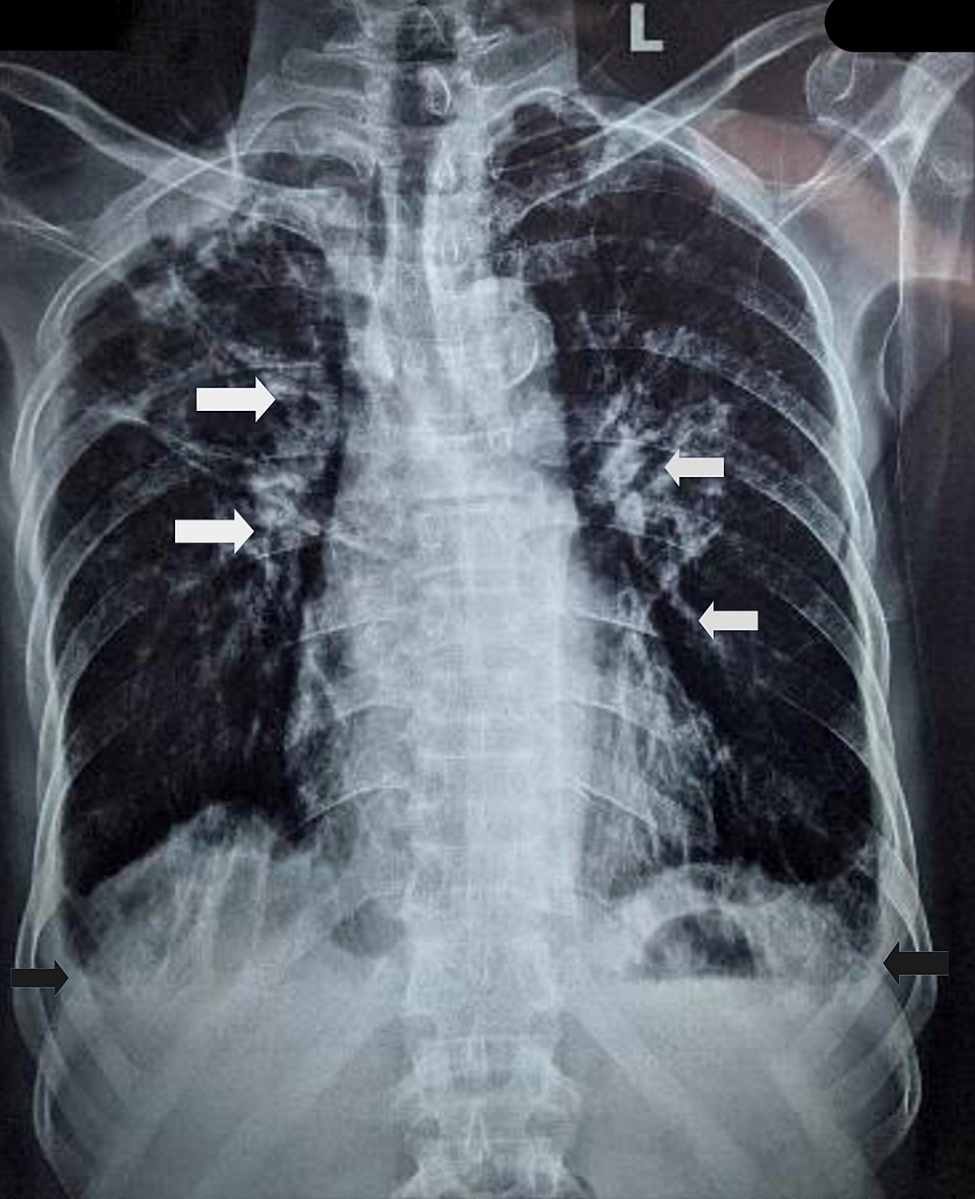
Chest X-ray PA view Few patchy areas of ground-glass opacities in B/L lungs; mild B/L pleural effusions (black arrow); calcified mediastinal and hilar lymphadenopathy (white arrow) PA: posteroanterior; B/L: bilateral
